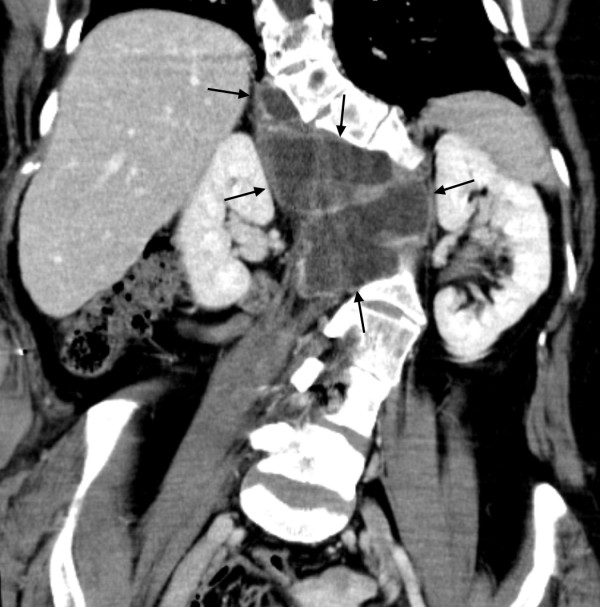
A 19 year-old female (Patient #1) with enlarging left plexiform neurofibroma which transformed to a malignant peripheral nerve sheath tumor. *A*. Plain radiograph shows a large left neck plexiform neurofibroma (large arrows) and suggests destruction of the posterior elements at C7 (small arrows). *B*. Coronal T1 without contrast shows the large left plexiform neurofibroma (arrows) with poor delineation of the bony elements. *C*. Axial MDCT without contrast demonstrates destruction of the T1 vertebral body, lamina and transverse process as well as the T1 rib (arrows). *D*. 3D volume rendered images with destruction of the bony structures at the C7 and T1 vertebral bodies (black arrows) and adjacent left T1 rib (white arrows).
